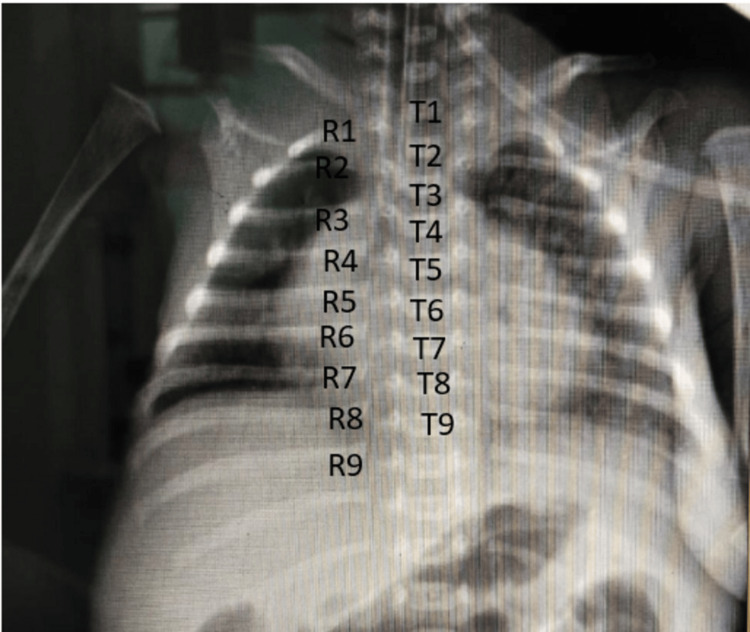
CXR image identification of vertebral levels for ETT tip position evaluation. CXR: chest x-ray, ETT: endotracheal tube
